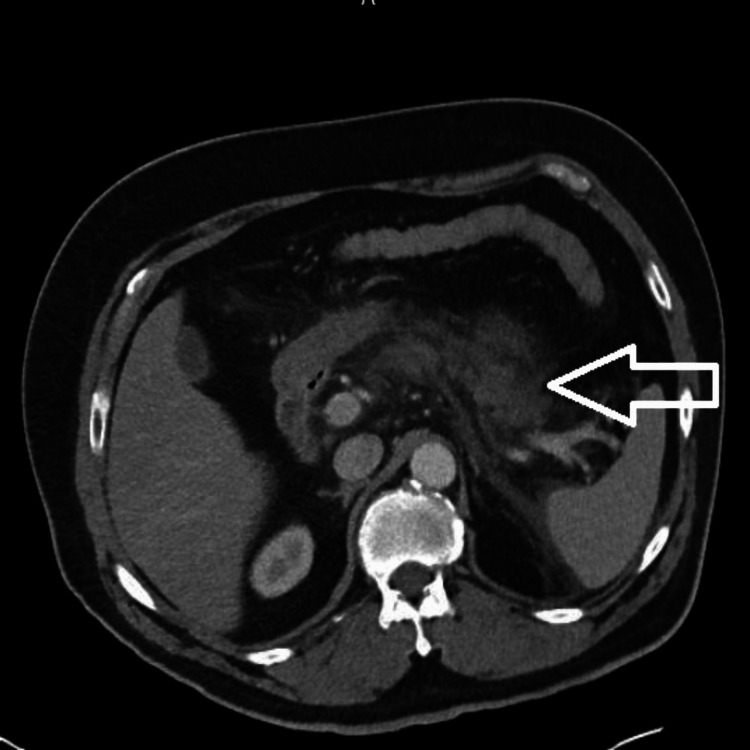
CT abdomen/pelvis performed at ED on arrival revealing extensive and diffuse peripancreatic stranding with surrounding fluid, which extends into the splenic hilum and along the undersurface of the spleen, as well as throughout the left pararenal space The arrow points towards acute pancreatitis. ED: Emergency department
